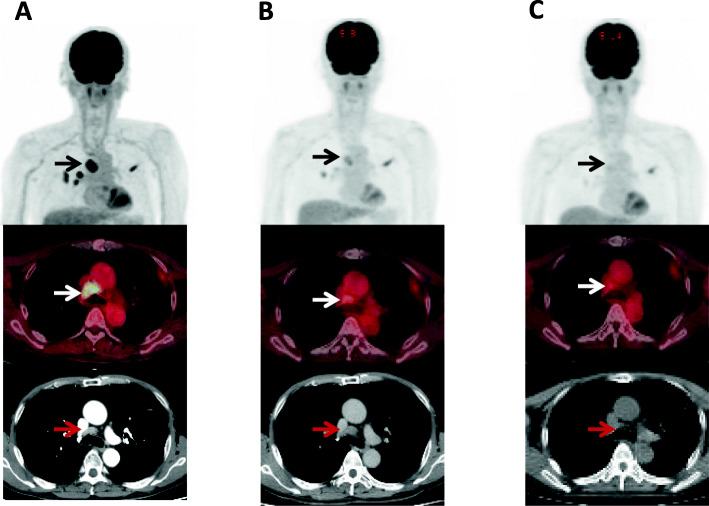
Therapeutic monitoring of ^18^F-FDG-PET in 71-years old male with advanced pulmonary adenocarcinoma who received pembrolizumab monotherapy as 1st line treatment. a
